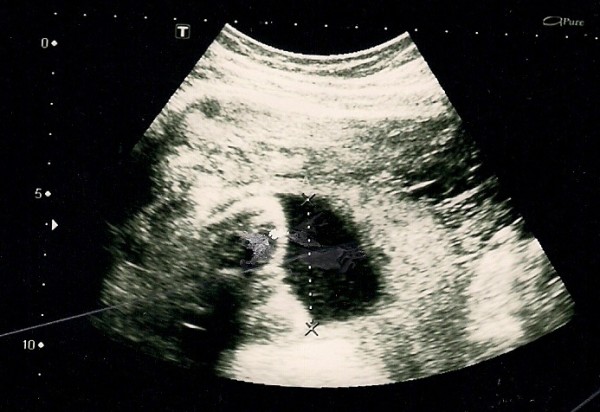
Ultrasonography picture at 23 weeks showing fetus, amniotic fluid and normal fetal morphology.
